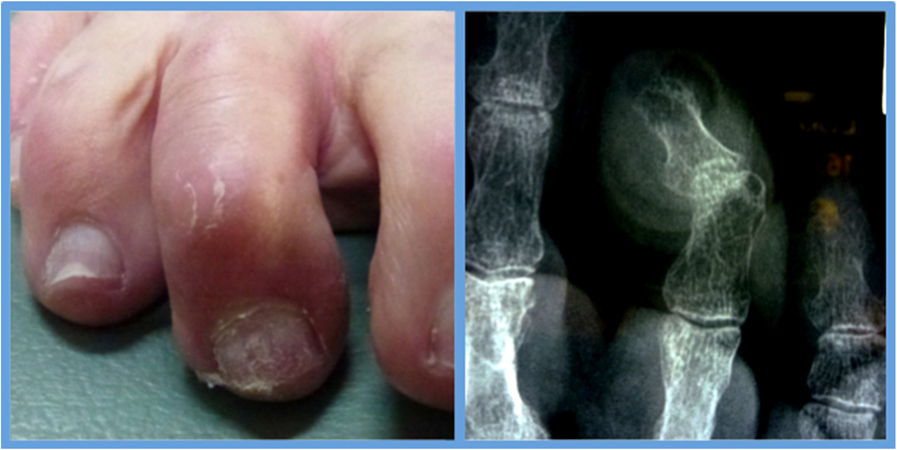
Simple digital deformity (pre-operative). Flexion deformity is seen at the distal interphalangeal joint of the right 3^rd^ toe clinically and radiographically.
